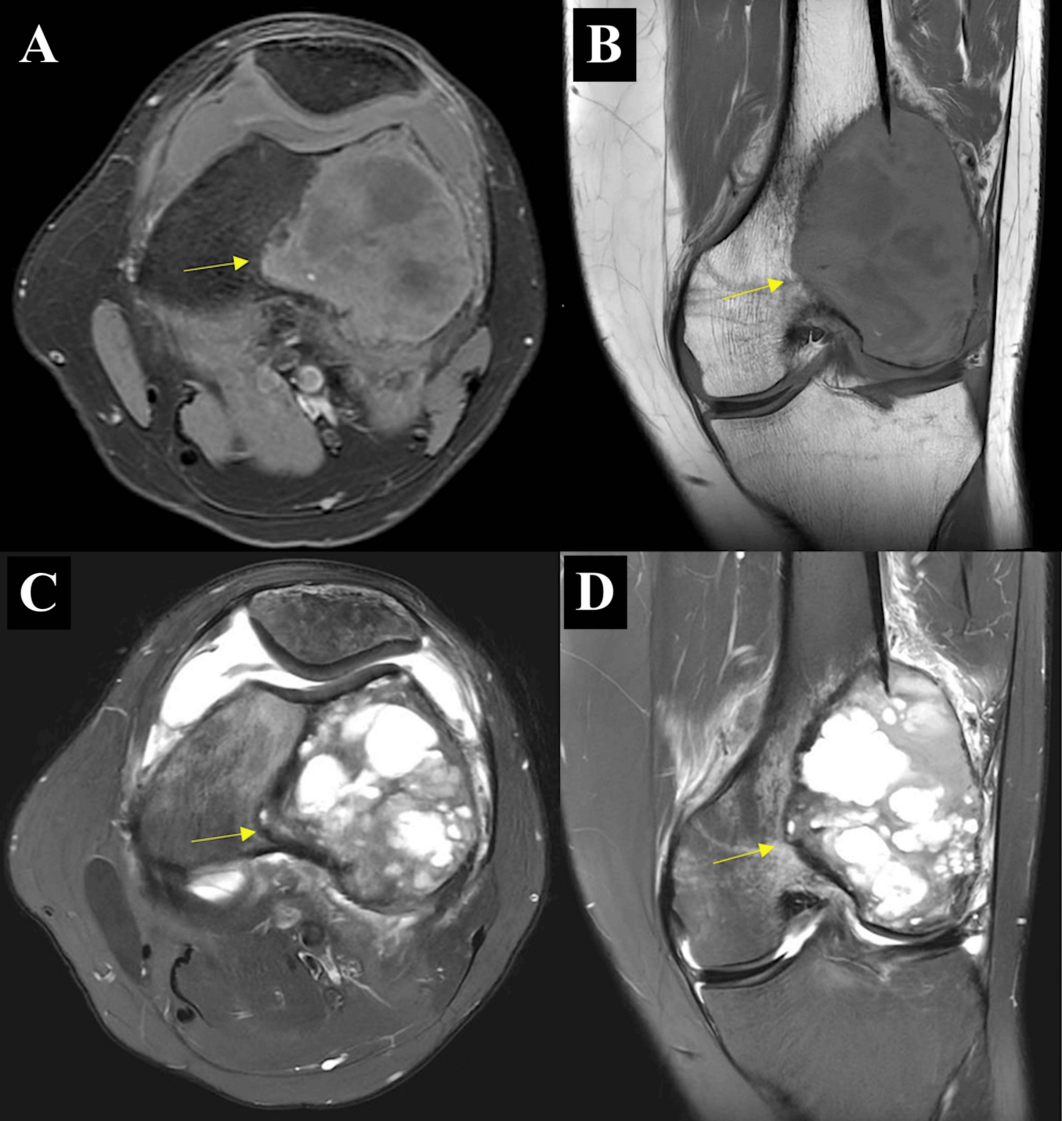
MRI images of the left distal femur T1 and T2 MRI images showing a lesion over the lateral femoral condyle with solid and cystic components, demonstrating low to intermediate T1-weighted signal and high to intermediate T2-weighted signal. (A) T1-weighted axial view; (B) T1-weighted coronal view; (C) T2-weighted axial view; (D) T2-weighted coronal view
